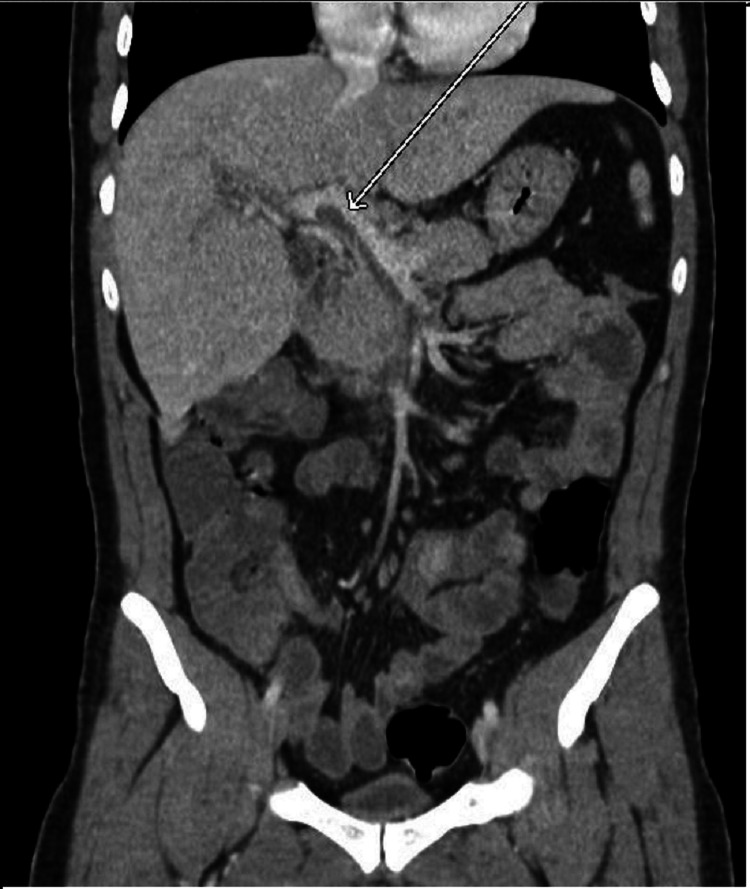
CT abdomen (coronal view) showing portal vein thrombosis
